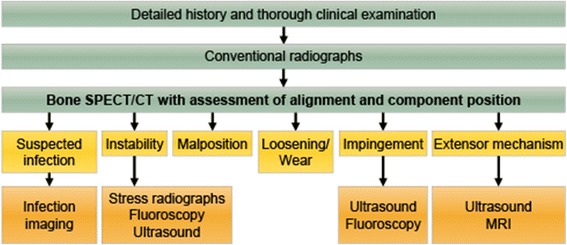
Imaging algorithm painful knee arthroplasty. Imaging algorithm for assessing the painful knee arthroplasty using bone SPECT/CT as second-line modality after conventional radiographs. Depending on the differential diagnosis generated by SPECT/CT selected follow-up imaging techniques may be required. Modified from Hirschmann et al. (Hirschmann et al. 2013)
